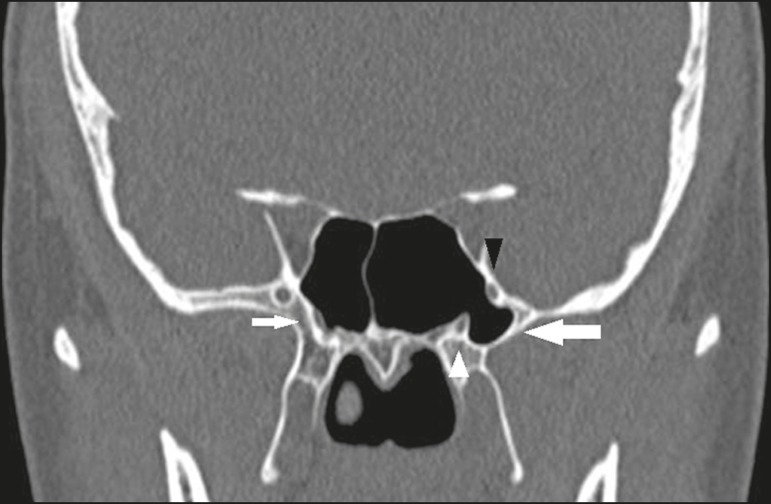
Coronal CT slice, with bone window settings, showing the left pterygoid
